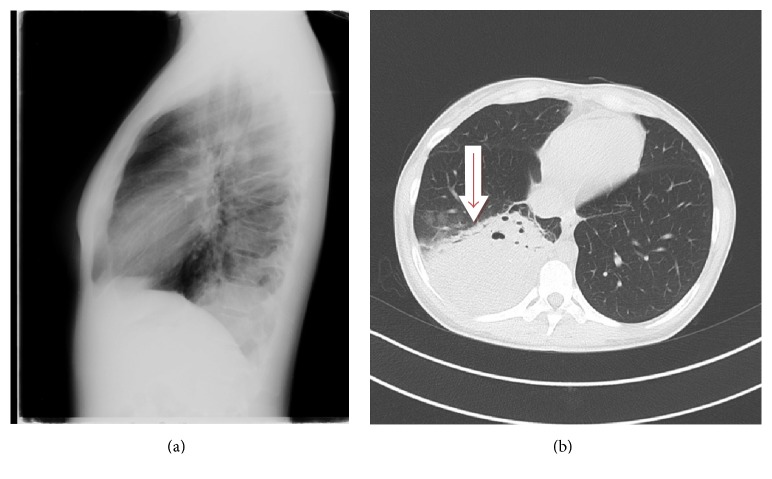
(a) Chest radiograph on presentation; (b) chest CT scanning on day 3 of admission, showing a large abscess in the right lower lobe.
